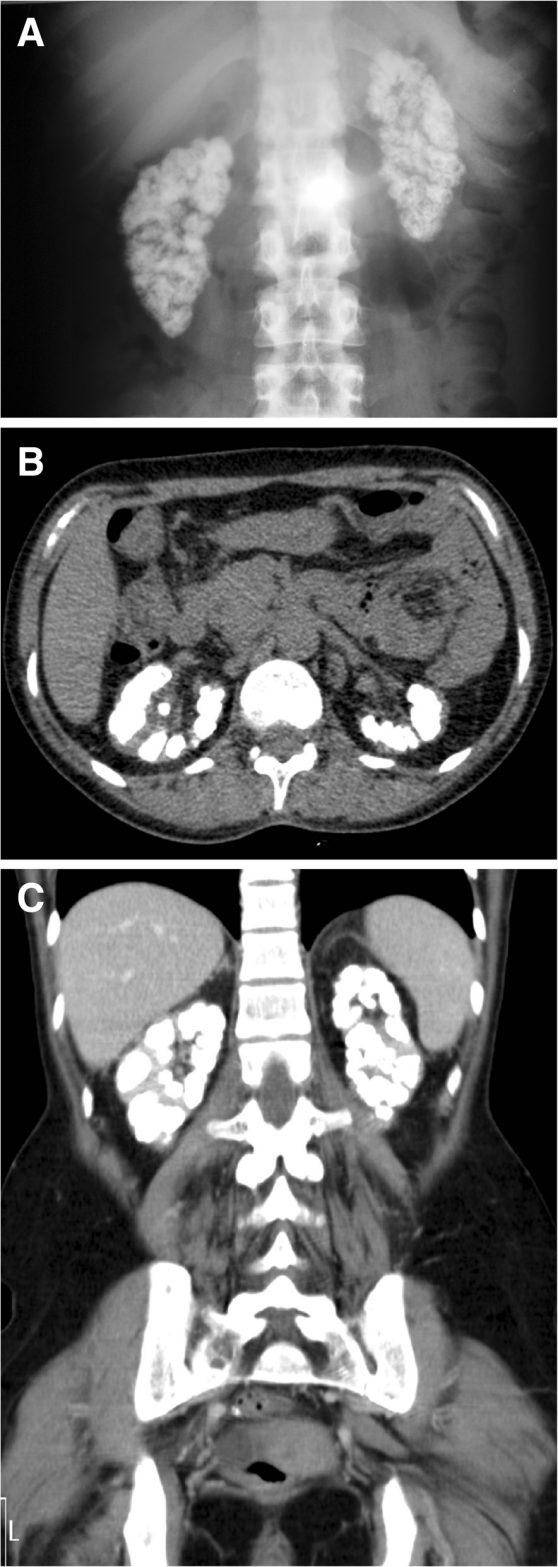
Calcifications visible in the abdomen of patient 1. Images reveal mixed nephrocalcinosis with accentuated calcifications in the renal cortex and medulla. (A) X-ray. (B) Computed tomography (longitudinal cut). (C) Computed tomography (coronal cut).
